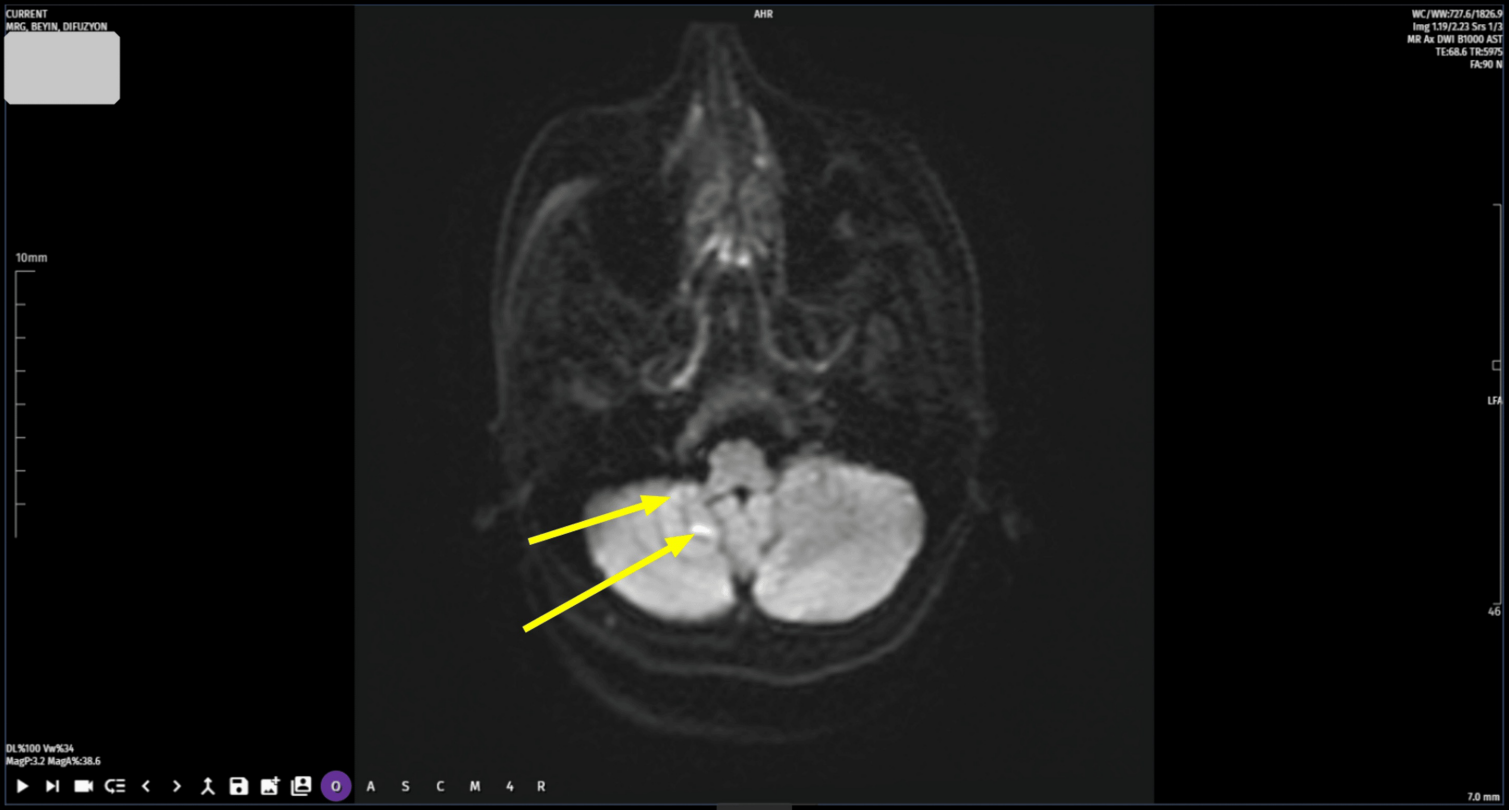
Diffusion-weighted MRI of the brain The arrows point to the hyperintense signals in the right cerebellar hemisphere and bulbar region.
